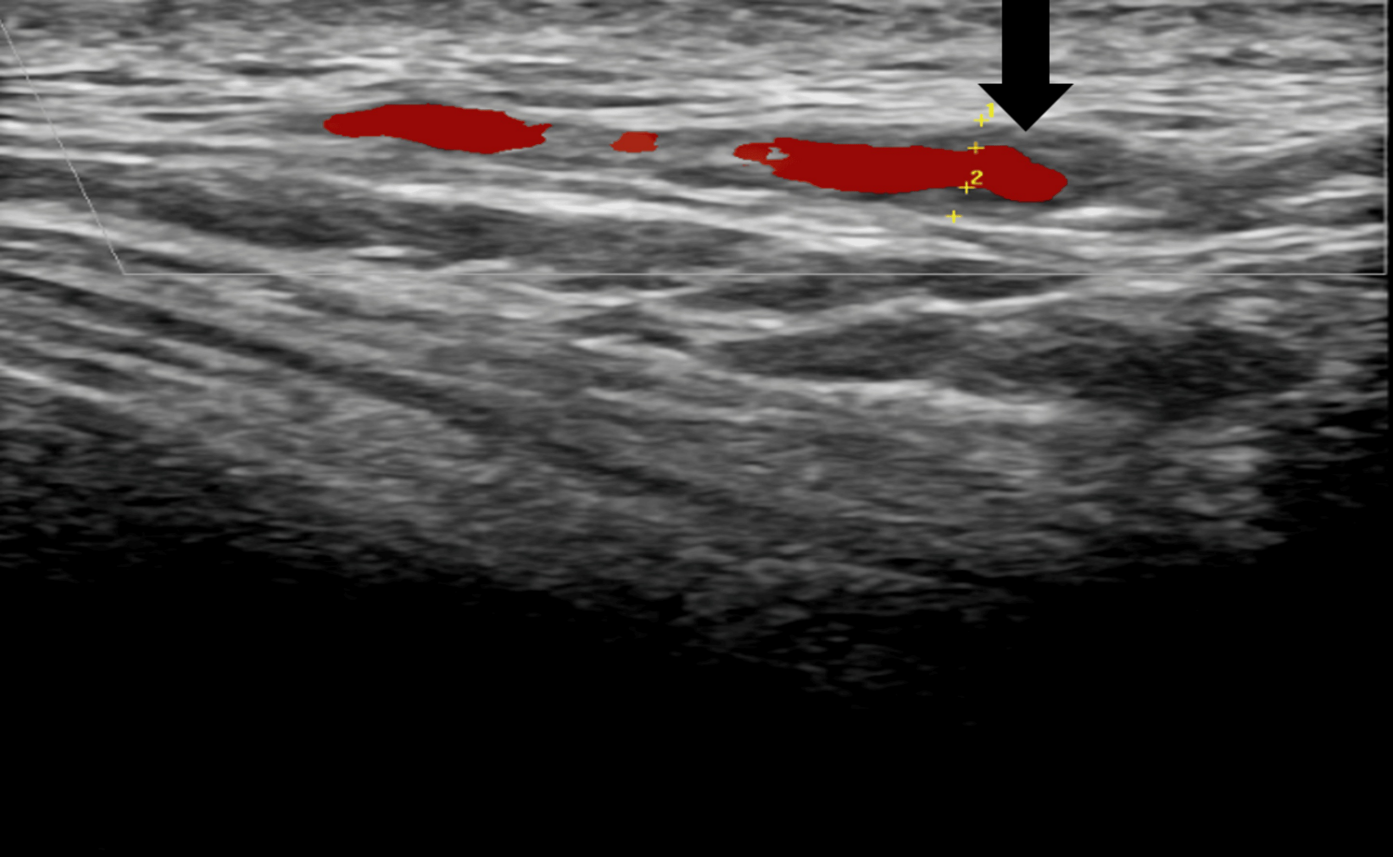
Halo sign seen in the distal temporal artery (marked) in the carotid artery duplex ultrasound
